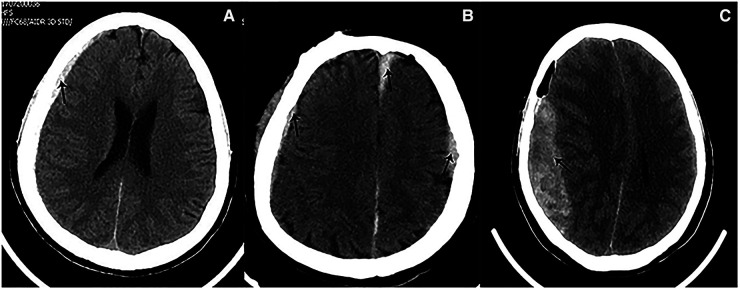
Postoperative imaging of patients with SDH. (A) Postoperative CT in a patient with left HFS showed a right frontal SDH (black arrow). (B) Postoperative CT in a patient with left HFS showed multiple sites of SDH (black arrow), involving bilateral temporal lobes and the cerebral falx. (C) Postoperative CT on a patient with right TN showed that a massive right temporal SDH (black arrow) lead to the displacement of the midline.
